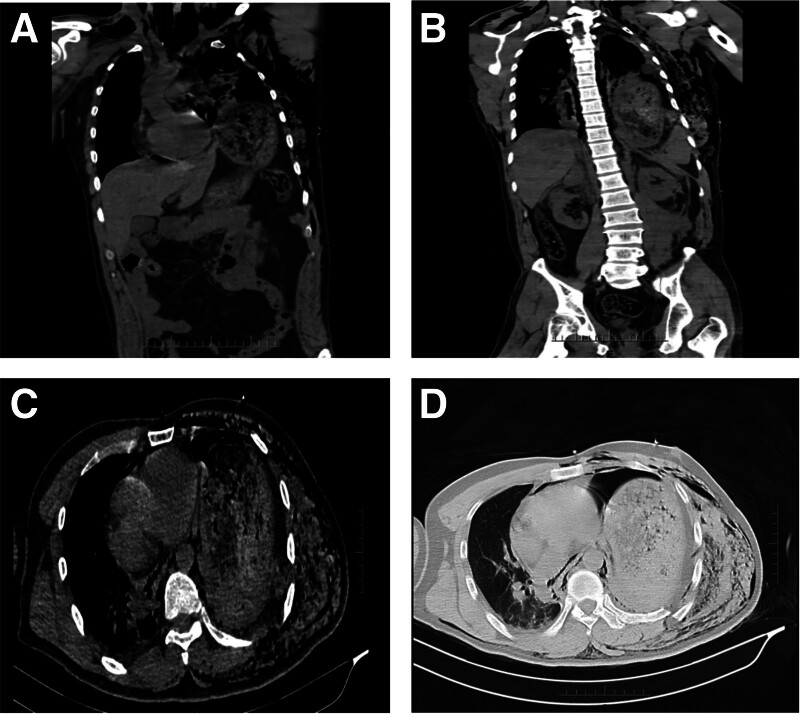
Patient’s chest and abdominal CT. (A) Stomach herniation into the left thoracic cavity. (B) Stomach and spleen above the diaphragm. (C) Left-sided tension viscerothorax with mediastinal and cardiac rightward shift. (D) Left-sided tension viscerothorax, mediastinal and cardiac rightward shift, left rib fractures, and significant left lung compression. CT = computed tomography.
